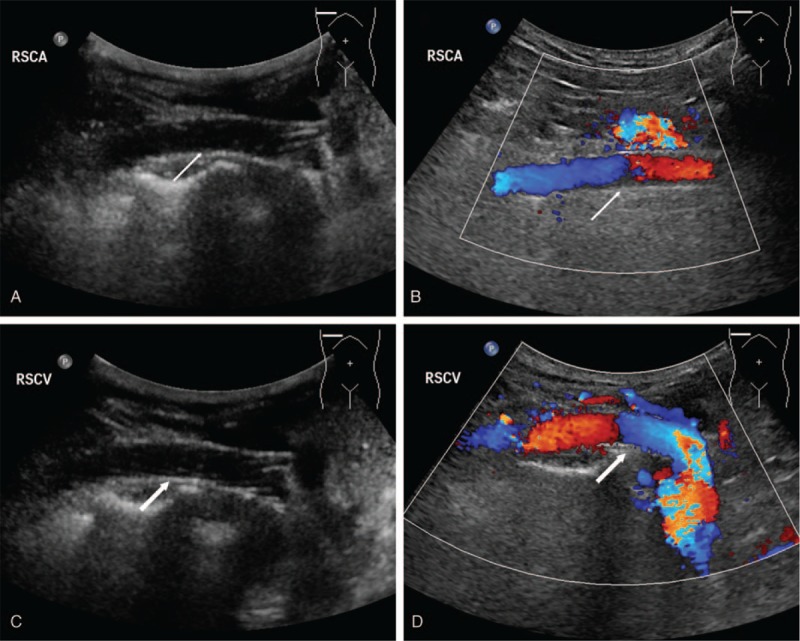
Ultrasonography image during 6-month follow-up showed the patent stent in the right subclavian artery (thin arrows in A and B) and the patent stent in the right subclavian vein (thick arrows in C and D).
